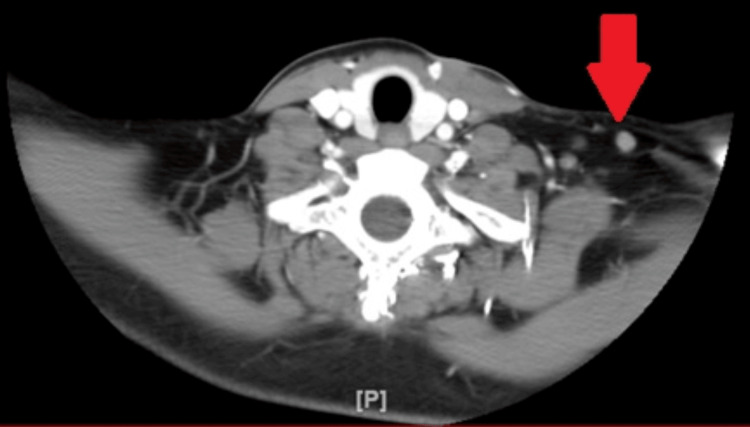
Computer tomography (CT) scan axial section of the neck and soft tissues showing supraclavicular lymphadenopathy (Red arrow)
